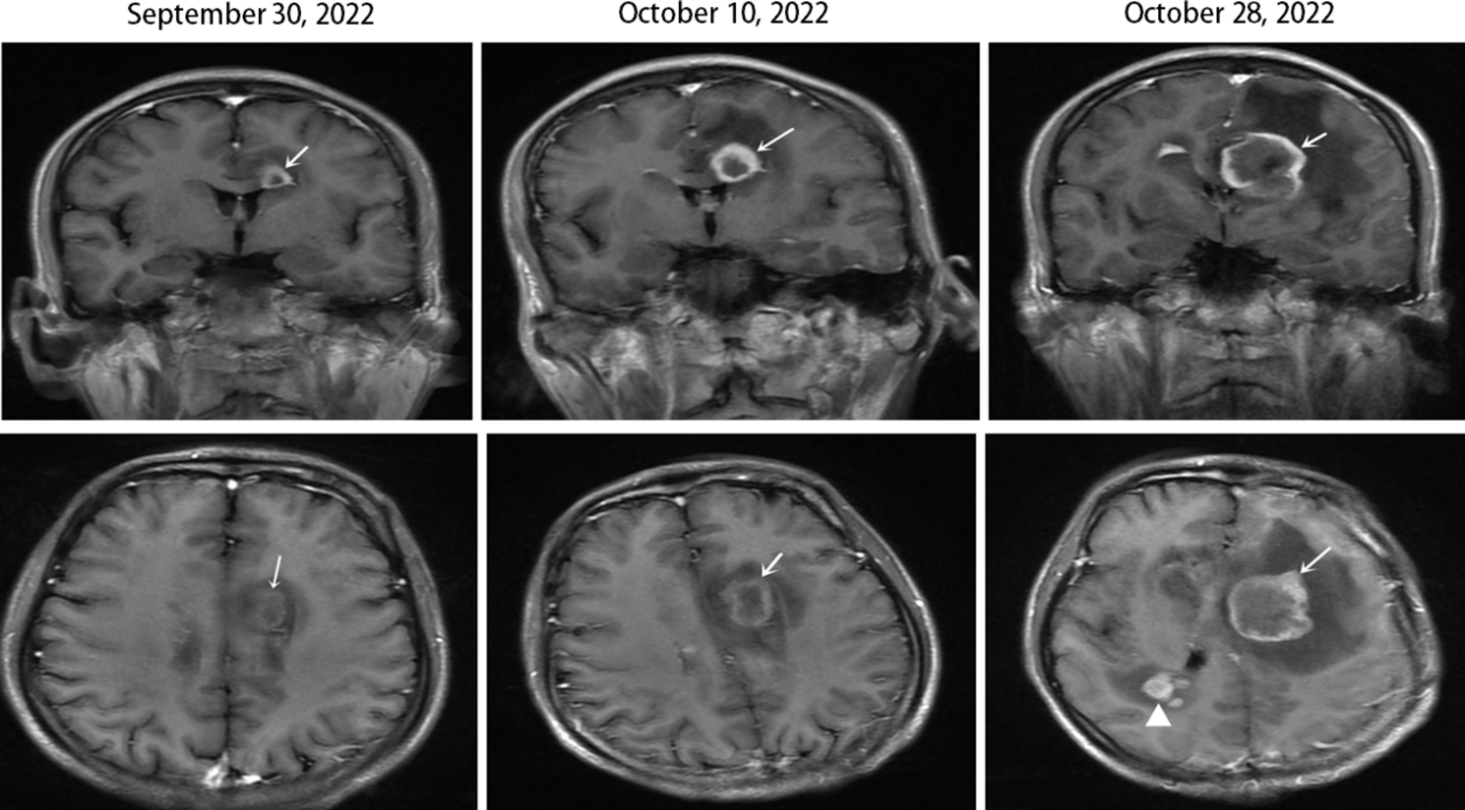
MRI of the brain of our case. The upper row are coronal-enhanced images and the lower row are axial-enhanced images. We can see the ring strengthening lesion in the left lateral ventricle para frontal angle involving the callosum (white → is the strengthening lesion) with central necrosis and perilesional edema. Both MRIs on October 10 and October 28 show progressive enlargement of the lesion with worsening of perilesional edema, and the white △ arrow shows a new lesion in the right lateral ventricle of the right parietal lobe
